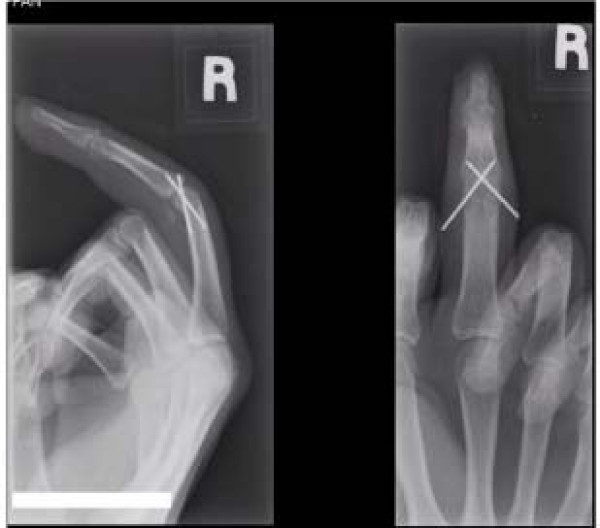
Post operative x-rays showing the reduced PIP joint and stabilized with K wires.
